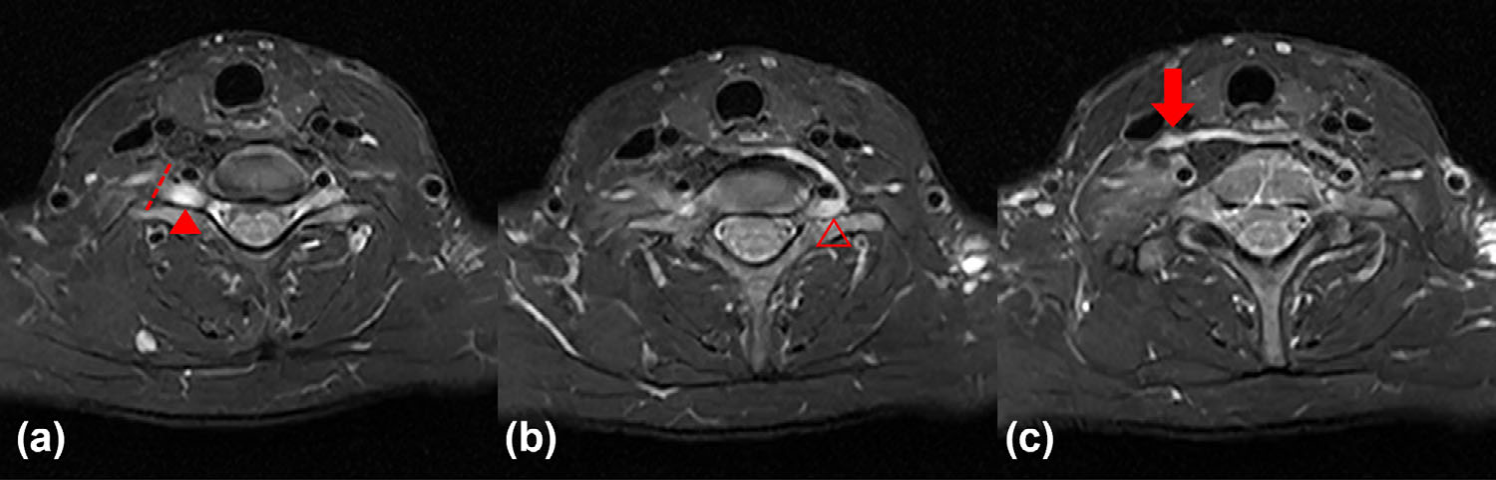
Axial T2‐weighted magnetic resonance imaging (MRI) images of the patient after CC7 surgery in different cross‐section. (a) Dashed line indicates the section position of the C7 nerve root on the paralyzed side near the intervertebral foramen, solid triangle represents the paralyzed side's dorsal root ganglia (DRG). (b) Hollow triangle represents the nonparalyzed side's DRG. It can be observed that the DRG on the affected side has a significantly increased volume and MRI signal intensity compared to the DRG on the healthy side. (c) Arrow indicates the site of nerve anastomosis after CC7 surgery.
